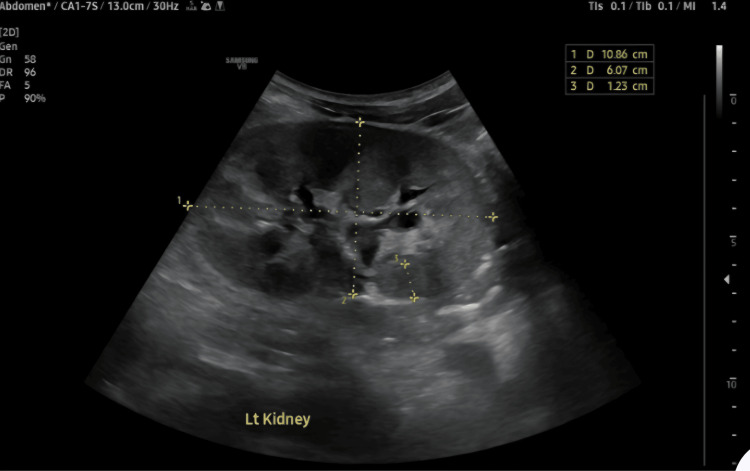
Ultrasonography of the left kidney showing increased cortical echogenicity and mild impairment of corticomedullary differentiation
